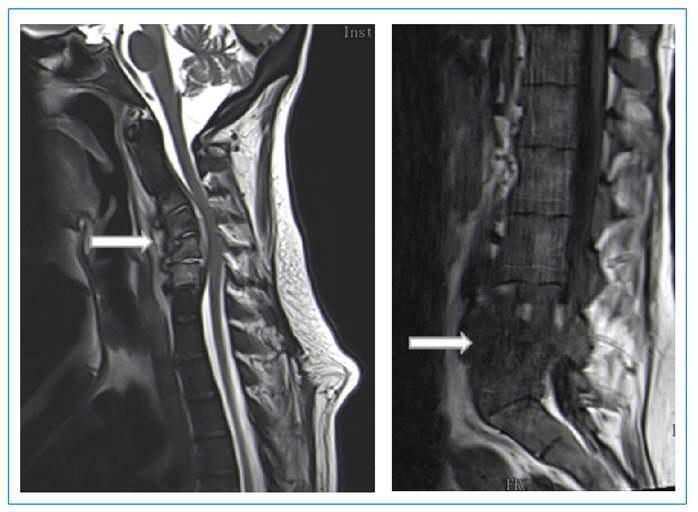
Magnetic resonance imaging of C3-C6 spondylodiscitis, spinal cord
